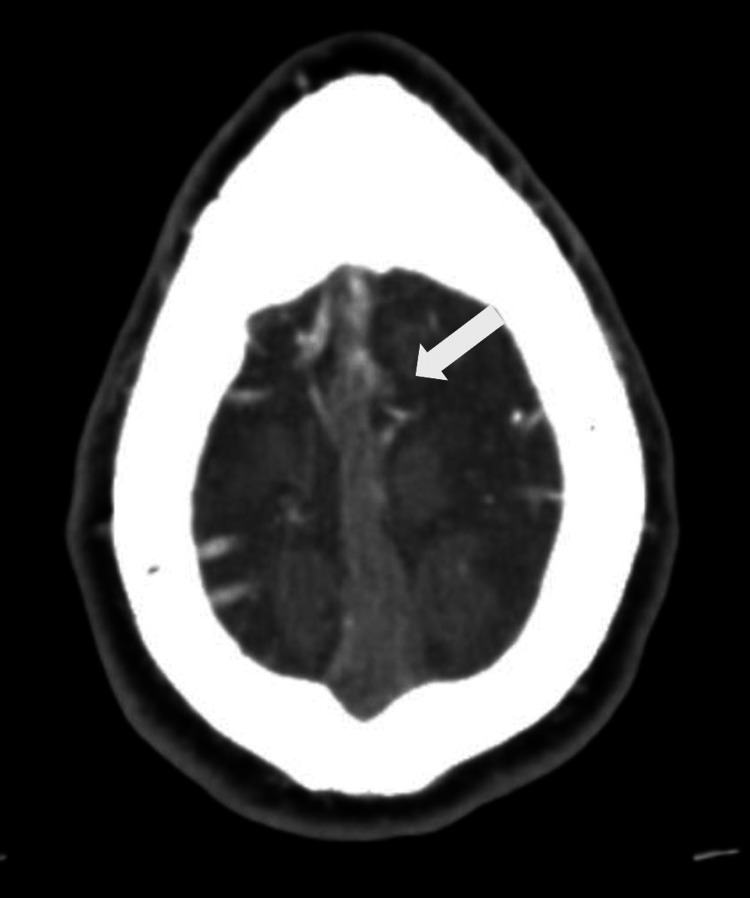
CT venogram demonstrating thrombosis of superior sagittal sinus (white arrow)
